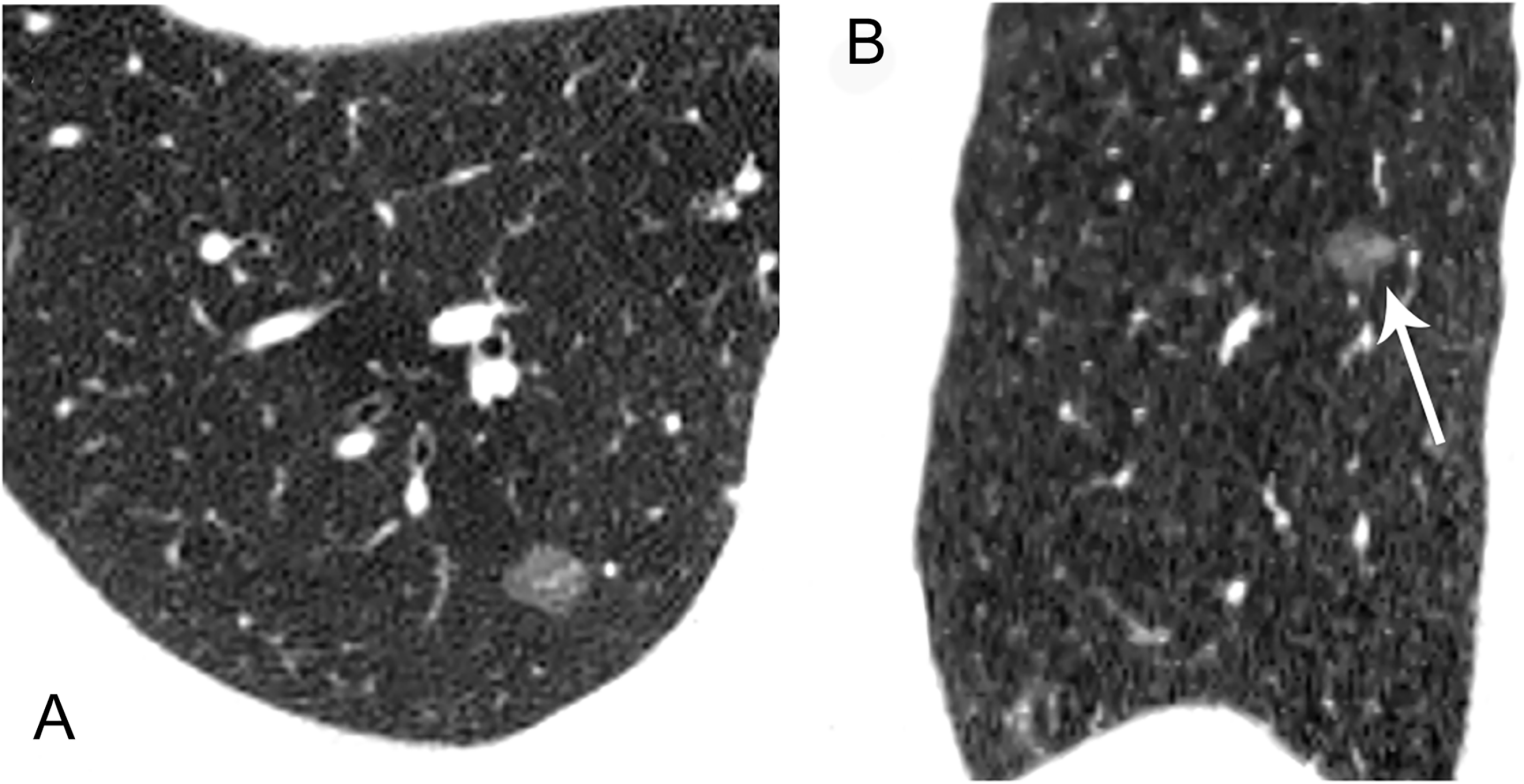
A 10mm pure GGN in axial image (A) of the lower lobe of right lung in a 62-year-old woman, sagittal (B) HRCT image shows an oval nodule with a notched border (arrow), and vessels passing through the pure GGN with tiny branches.
